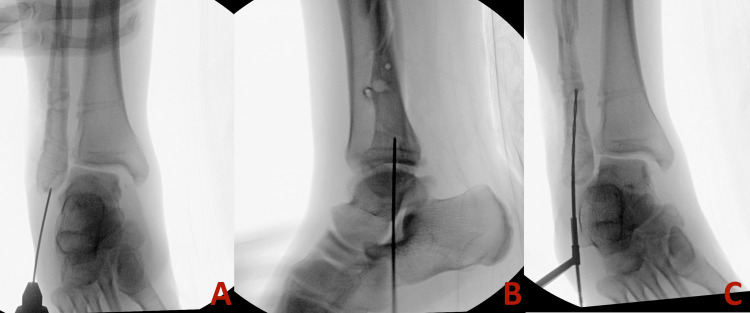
Intraoperative radiographs depicting guidewire placement impinged on medial fibula cortex preventing further advancement of the guidewire. A: Anterior-posterior view. B: Lateral view. C: Oblique view
